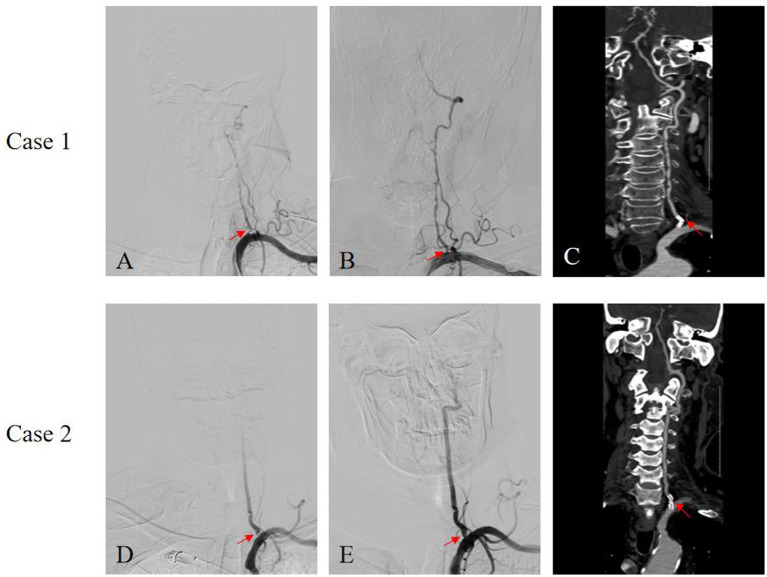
Stent fracture in two cases. (A) Pre-operative (Case 1); (B) Post-stenting (Case 1); (C) Six-month CTA(Case 1); (D) Pre-operative (Case 2); (E) Post-stenting (Case 2); (F) Six-month CTA (Case 2).
